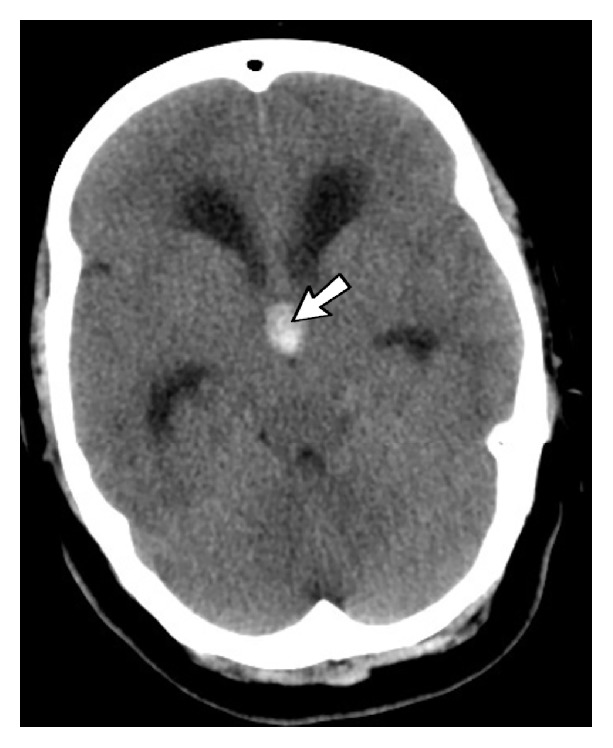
Axial noncontrast brain CT scan shows a hyperdense mass (arrow) at the location of interventricular foramen of Monro. Frontal and temporal horns of lateral ventricles are visible and enlarged.
